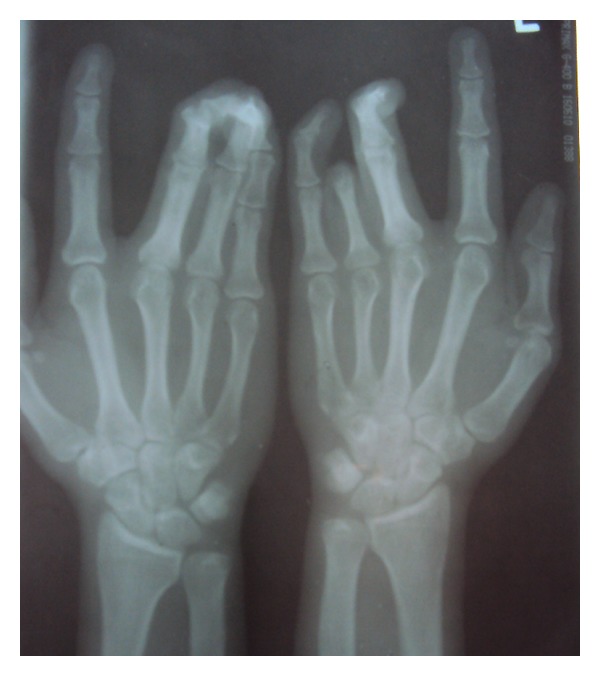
Hand wrist X-ray showing bony union of 3 fingers.
